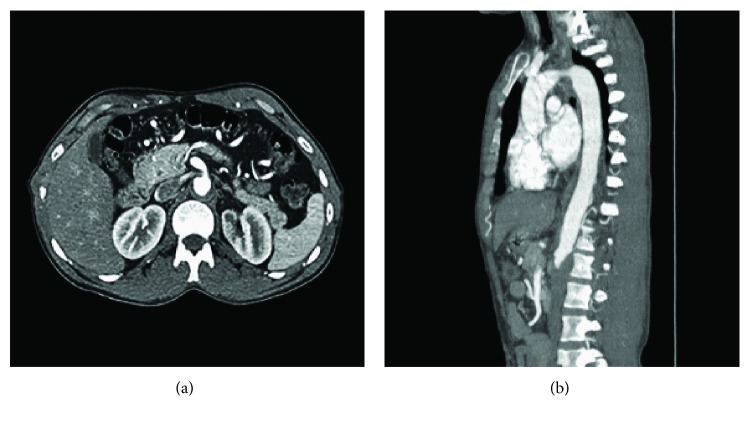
CT angiogram of the abdomen. (a) Bilateral stenosis and near occlusion of renal arteries. (b) Abdominal aortic thrombus that extends to the level of renal arteries.
